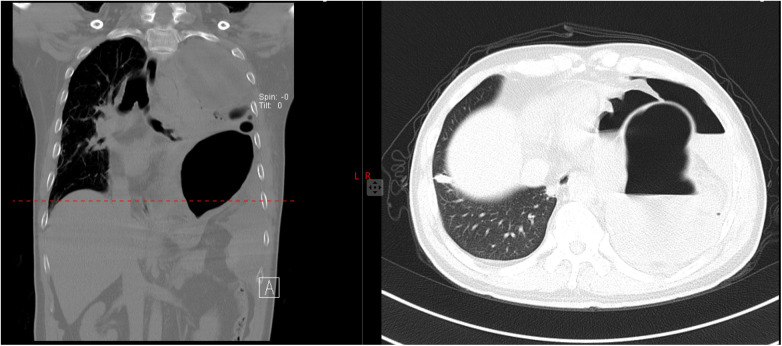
Chest CT plain scan: the left side is a coronal image, and the right side is an axial image (corresponding to the red dashed layer of the coronal position). The images show: a gas-containing gastric cavity is herniated into the left thoracic cavity (round-shaped hyperlucent shadow in the left thoracic cavity on the right axial image); the left lung tissue is compressed and collapsed (partial aggregation of lung markings can be seen in the right lung field); left pneumothorax is formed (a low-density area without lung markings in the left thoracic cavity), accompanied by mediastinal shift to the right.
